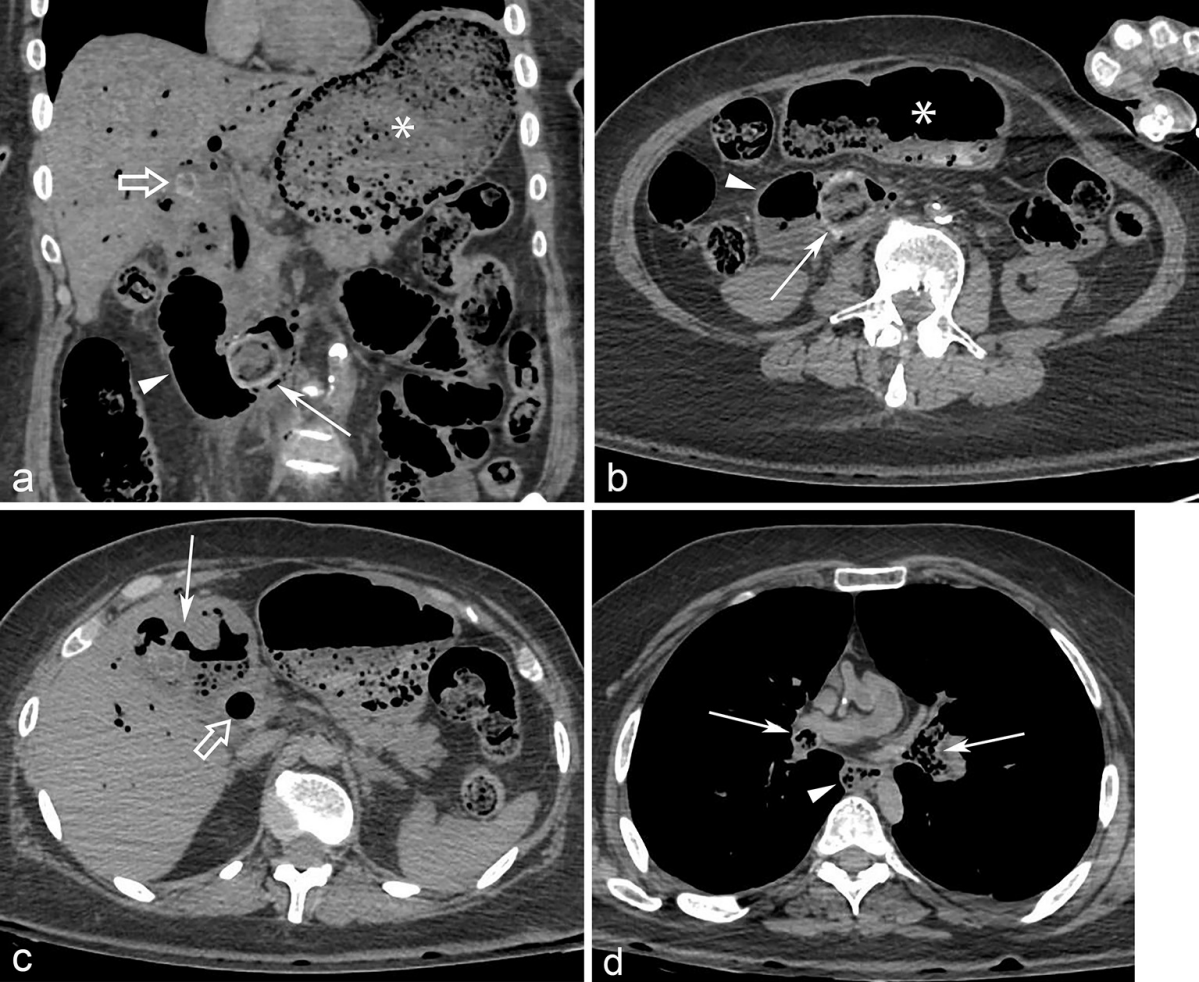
Post-mortem unenhanced CT in case of Bouveret’s syndrome. Coronal (a) and axial (b) unenhanced CT images through the level of the upper abdomen demonstrate a markedly distended stomach (asterisks) and proximal duodenum (arrowheads). A large peripherally calcified gallstone is seen impacted in the 4th part of the duodenum (arrow). Several other calcified calculi are also seen within the gallbladder (open arrow), with extensive pneumobilia throughout the intrahepatic biliary tree. Axial (c) image at the level of the gallbladder shows a gas and fluid communication (arrow) between the gallbladder fundus and adjacent duodenal bulb. The pancreatic portion of the common bile duct is dilated by gas (open arrow). Axial (d) image in the same patient at the level of the main bronchi demonstrates mixed density material throughout the central tracheobronchial tree (arrows). Debris extends into the second and third order branches (not shown), and is also seen filling the esophagus (arrowhead).
